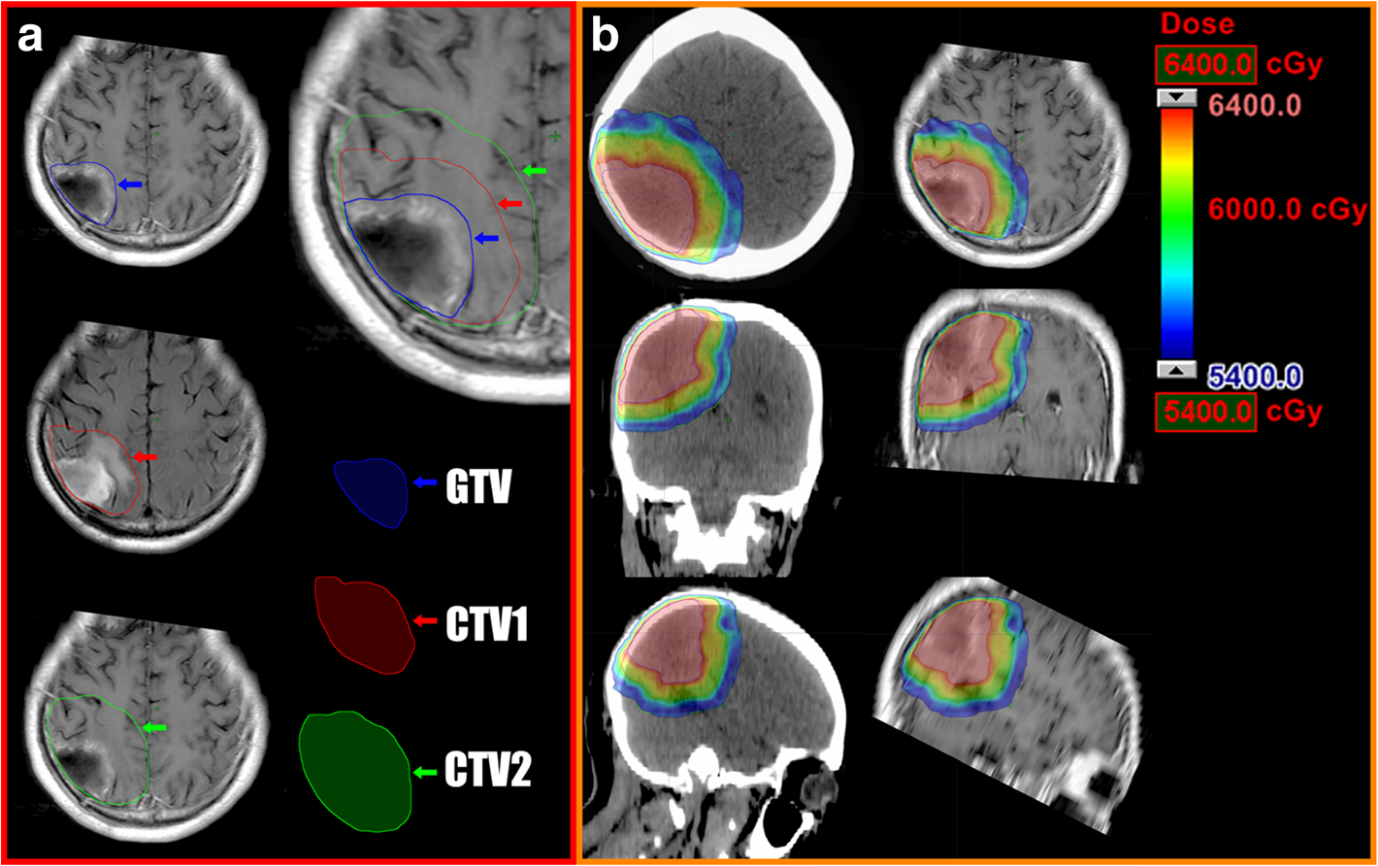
The target volume delineation and isodose distribution of a representative patient who received hypofractionated SIB-IMRT. a GTV is identified with a blue arrow, including the contrast-enhanced lesion on the postoperative T1-weighted MR images and the postoperative cavity; CTV1 is identified with a red arrow, including GTV plus a 1-cm margin and the surrounding edema on T2-weighted fluid-attenuated inversion recovery MRI; CTV2 is identified with a blue arrow, including CTV1 plus a 2-cm margin. b Isodose distribution in CT and MRI, showing the target volumes receiving 64 Gy (red), 60 Gy (green), or 54 Gy (blue)
